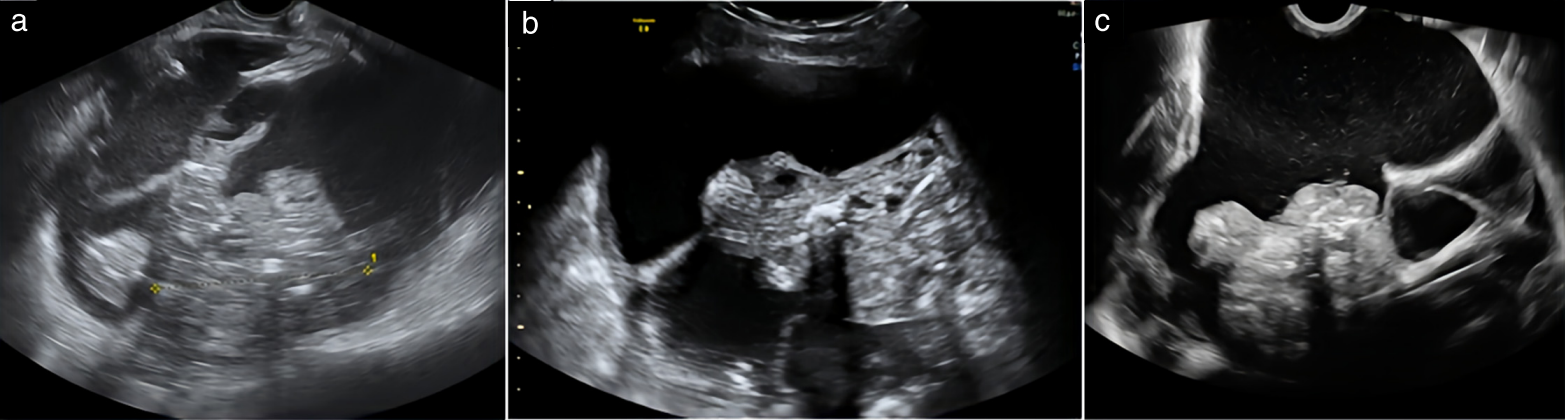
(a–c) Grayscale ultrasound images of three ovarian immature teratomas. Using pattern recognition, these tumors were described as multilocular‐solid tumors with solid tissue entrapped among septa. Solid components show pathognomonic echogenicity, with hyperechogenic areas, cysts and shadowing.
